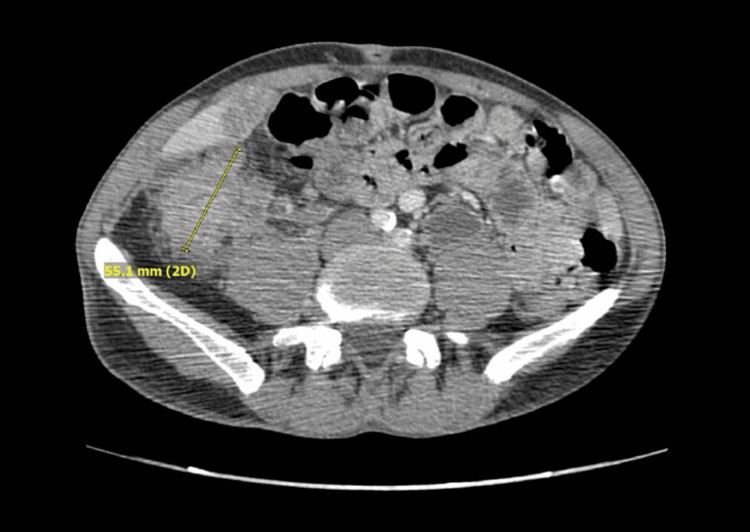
Initial CT abdomen showing a large right-sided colon mass, 06/4/6021
